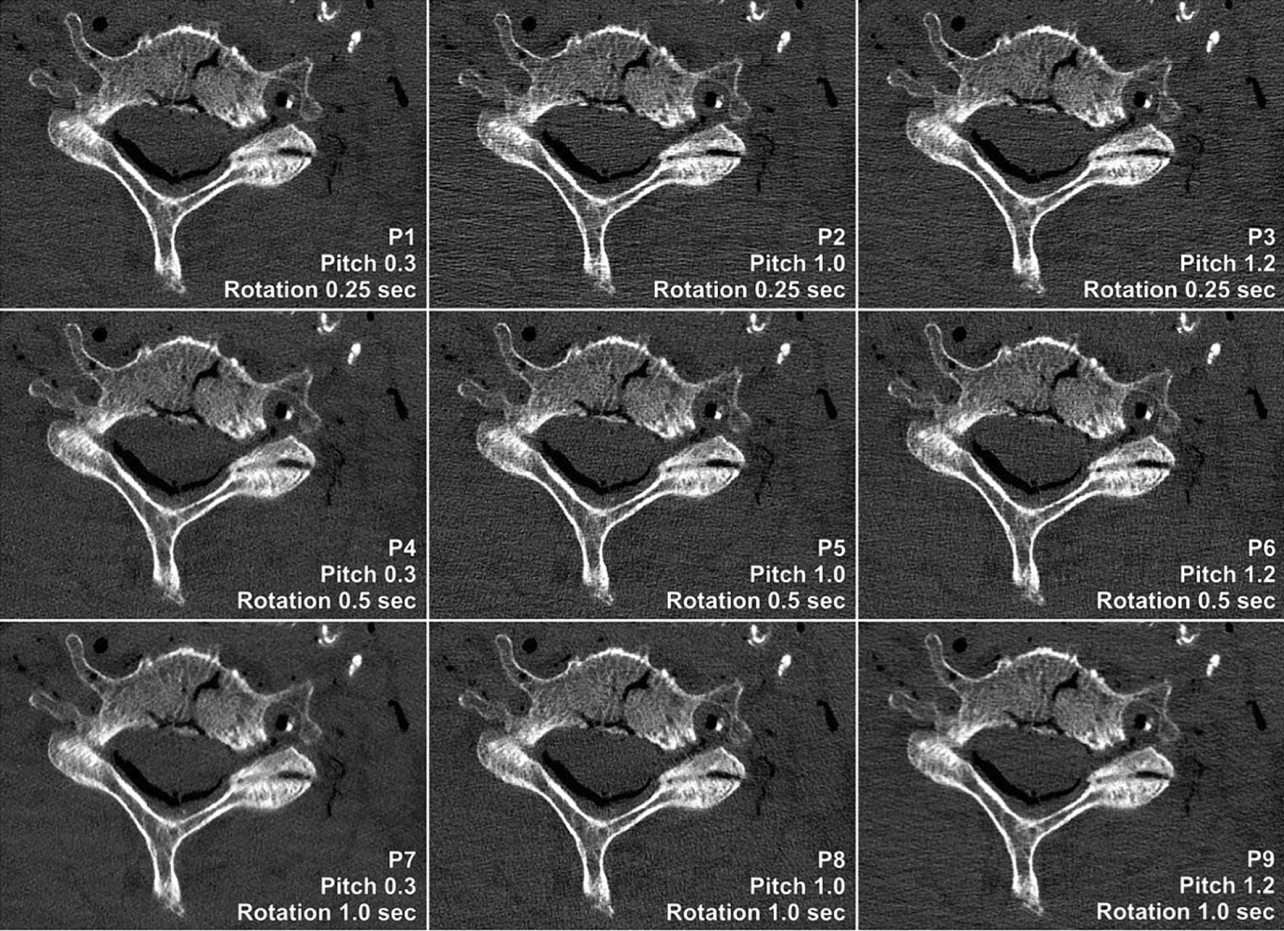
Ultrahigh-resolution photon-counting CT of the cervical spine with different combinations of helical pitch factor and gantry rotation time. Note the pronounced differences in image noise and sharpness among the nine scan protocols despite comparable radiation doses (5.71–5.94 mGy).
